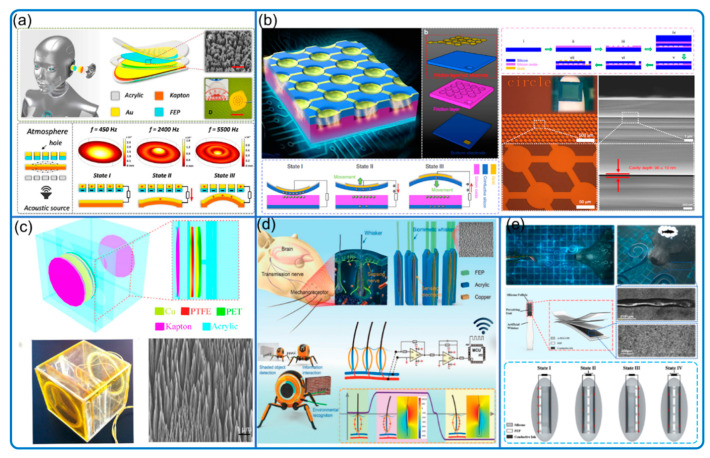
Remote media vibration sensor. (a) Auditory sensor. Reproduced with permission. Copyright 2018, AAAS [67]. (b) Micro triboelectric ultrasonic device. Reproduced with permission. Copyright 2020, American Chemical Society [69]. (c) Acoustic source locator in underwater environment. Reproduced with permission. Copyright 2015, Springer [70]. (d) Biomimetic hairy whiskers. Reproduced with permission. Copyright 2021, Wiley Online Library [71]. (e) Underwater bionic whisker sensor. Reproduced with permission. Copyright 2022, Elsevier [72].
